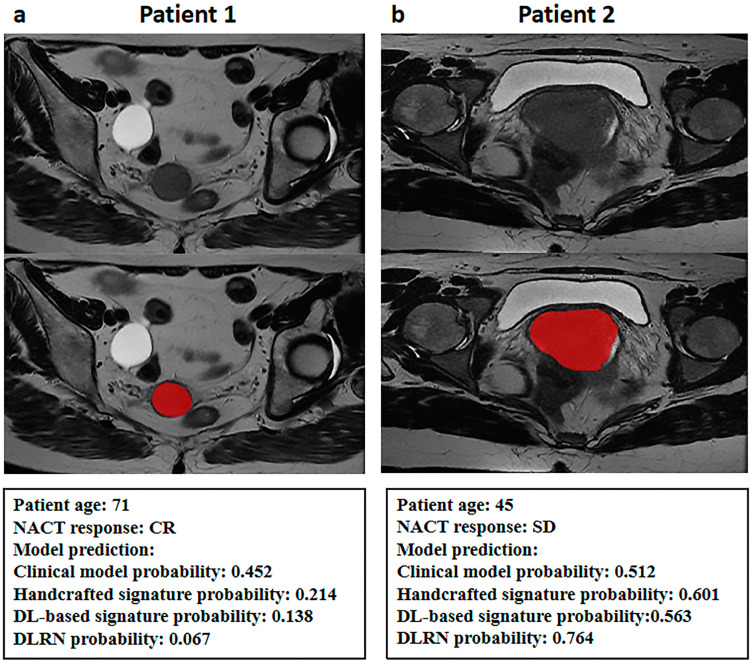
Two typical cases with MR images, clinical characteristics, and predicted results of response to NACT by different models. The red area represented the tumor issue. A 71-year-old woman (a) with complete response (CR). The probability of chemoresistance predicted by clinical model, handcrafted radiomics signature and DL-based signature was 0.452, 0.214 and 0.138, and that of chemoresistance predicted by the DLRN was 0.067. A 45-year-old woman (b) with stable disease (SD). The probability of chemoresistance predicted by clinical model, handcrafted radiomics signature and DL-based signature was 0.512, 0.601 and 0.5133, and that of chemoresistance predicted by the DLRN was 0.764. T2WI = T2-weighted imaging; DWI = diffusion-weighted imaging; CE-T1WI = contrast-enhanced-T1 weighted imaging.
